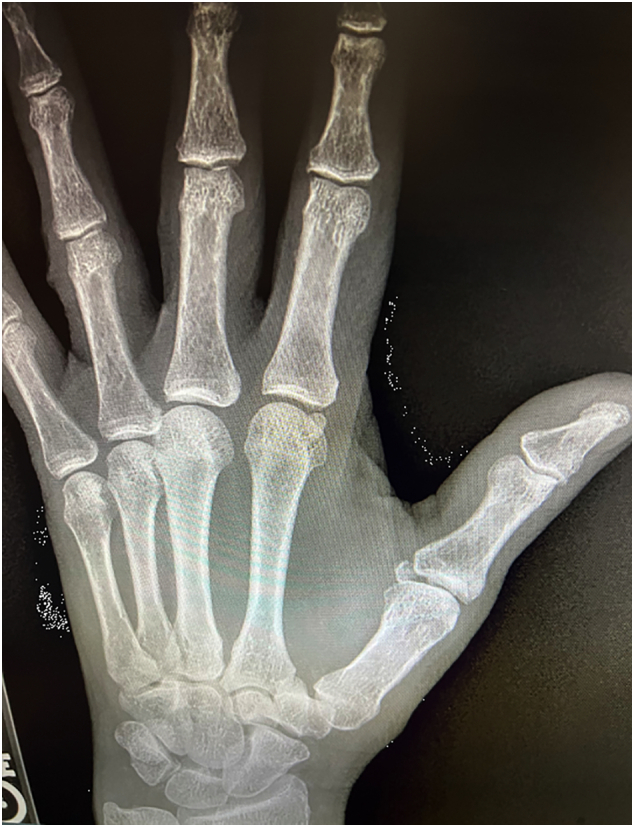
Radiographic image of hand.
